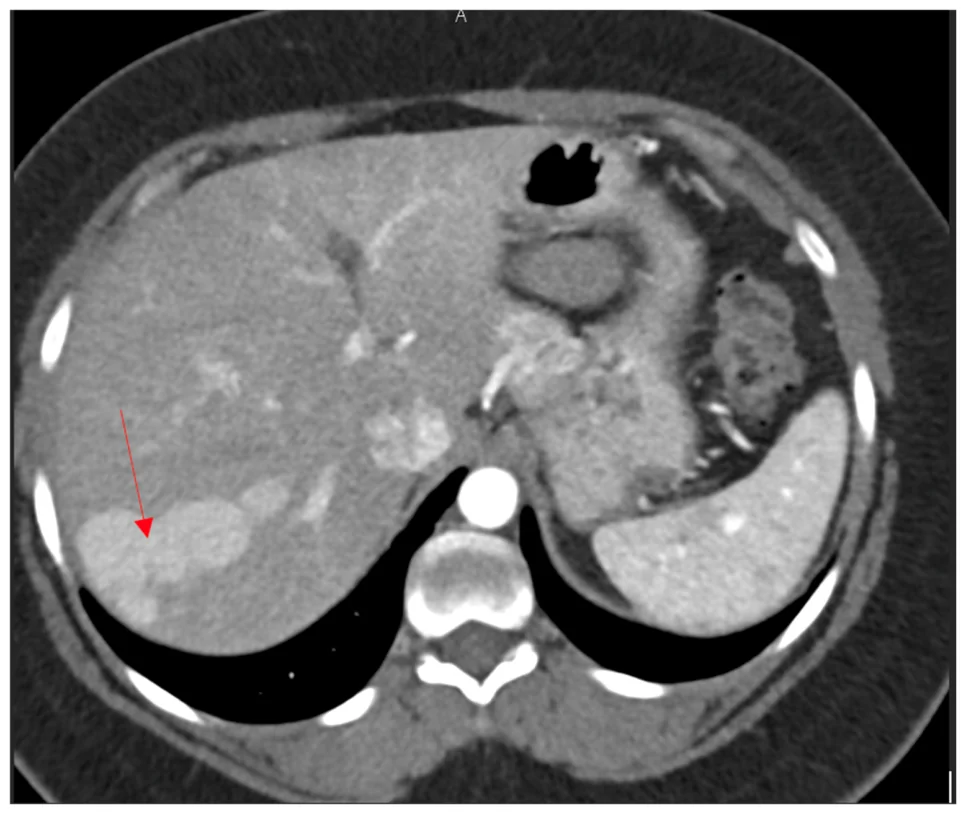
Axial CTA showing an intrahepatic portosystemic venous shunt (arrow) between the right portal and right hepatic veins.
